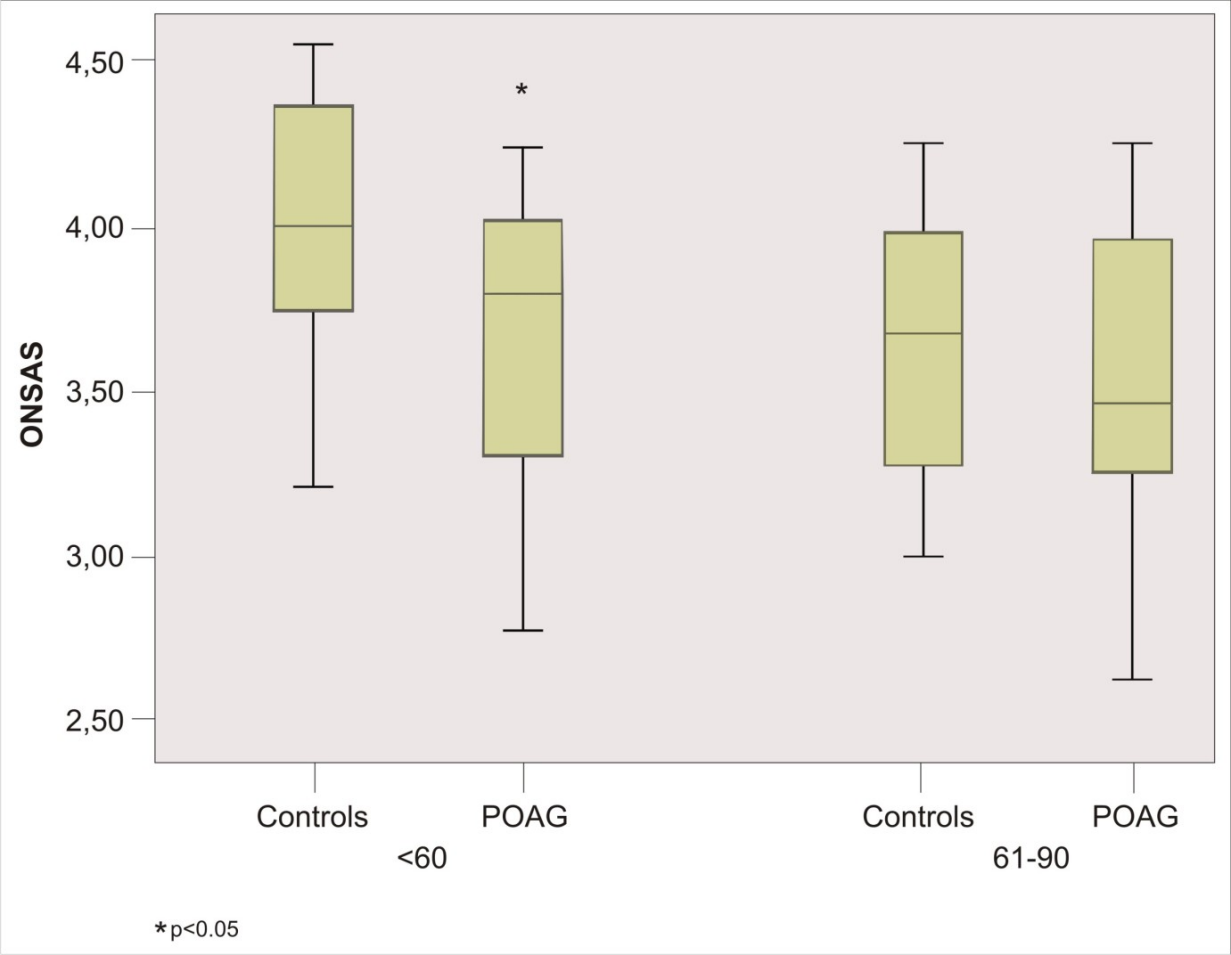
Box plots showing A-scan ultrasound measurements of the optic nerve subarachnoid space (ONSAS) in primary open angle glaucoma (POAG) and control groups that were compared according to age patient.
